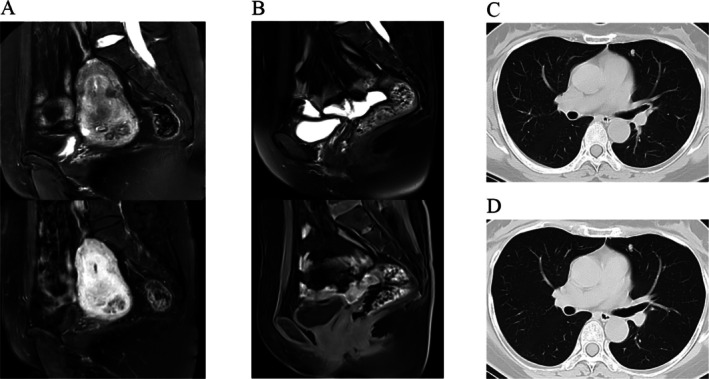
Imaging examination results before and after treatment. (A) Pelvic MRI revealed diffuse lesions within the uterine cavity and cervix, with the largest lesion measuring 7.6 cm in diameter. (B) The pelvic MRI demonstrated postoperative changes. (C) Chest CT revealed a solid nodule in the upper lobe of the left lung, with a maximum transverse diameter of 8 cm. (D) Chest CT again demonstrated that the size of the nodule was unchanged compared to earlier findings.
